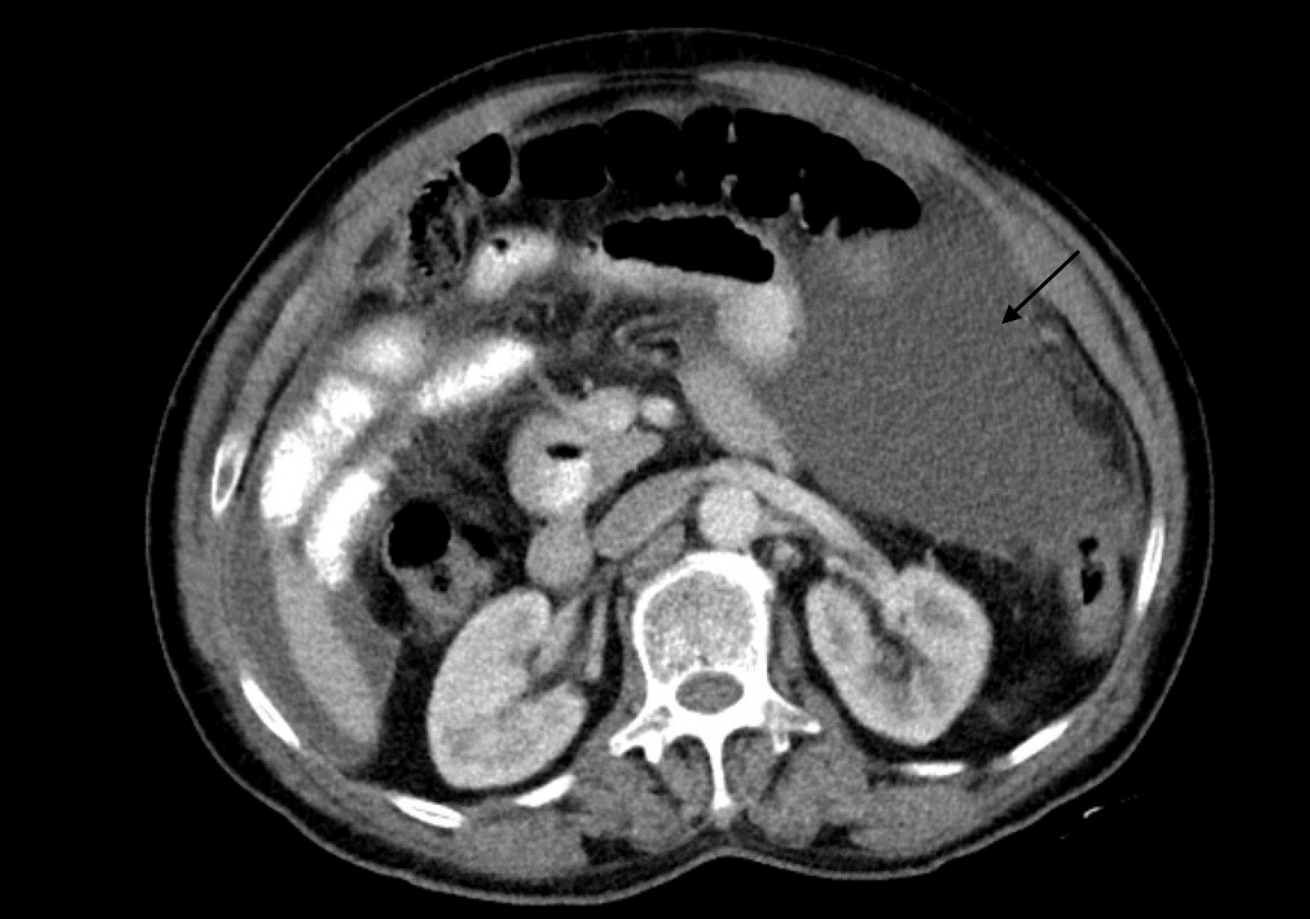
CT scan of the abdomen and pelvis revealing abdominal ascites (arrow)
